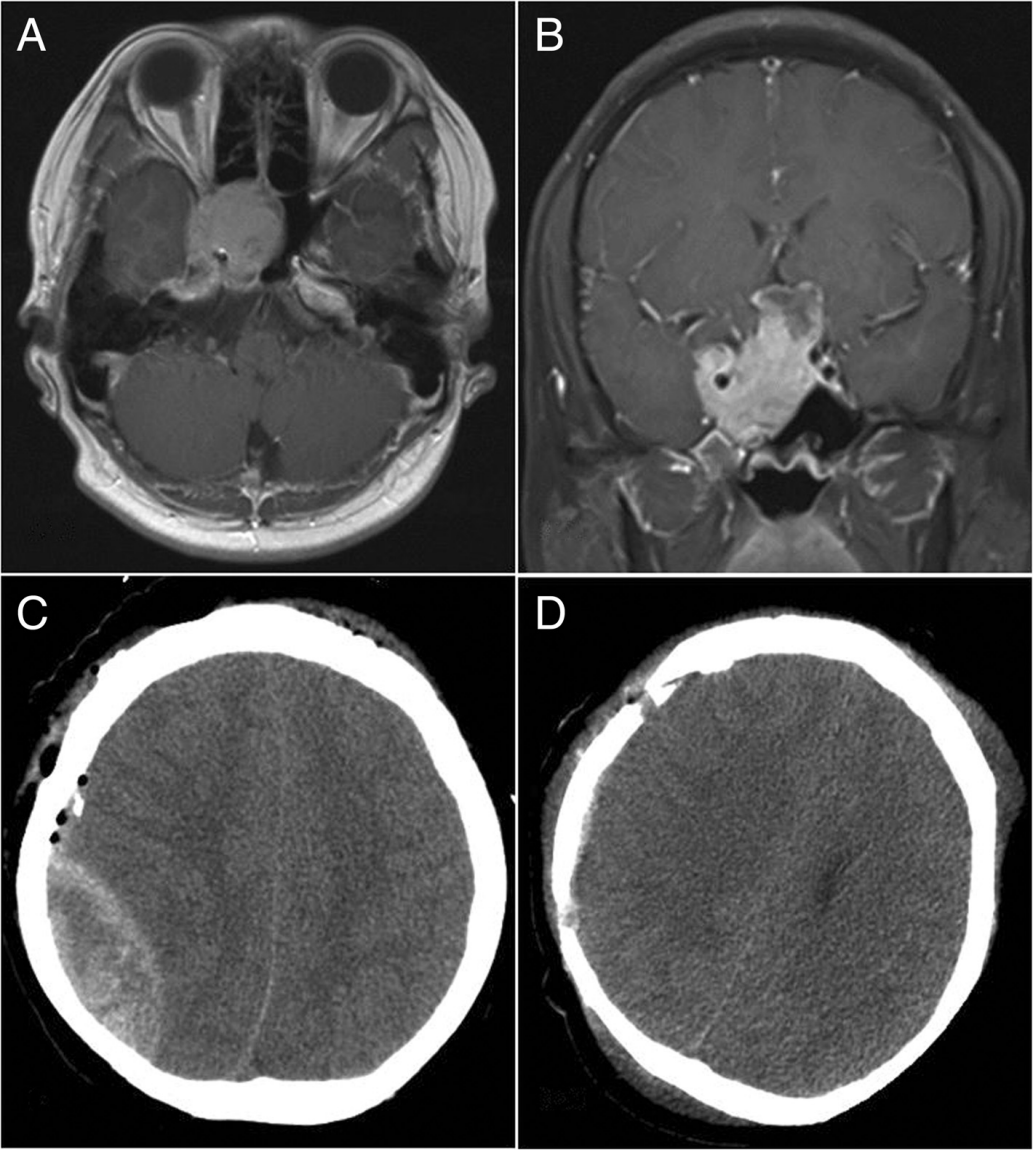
Ipsilateral remote hemorrhage in case 1. a, b Preoperative-enhanced magnetic resonance imaging (MRI) revealed a sellar tumor and normal ventricular size with no expansion. c CT revealed an epidural hematoma behind the surgical field after the tumor resection. d CT showed that the hematoma had been cleared
